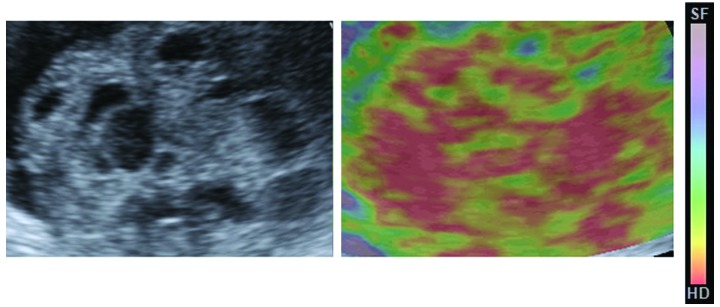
Conventional sonogram and elastogram from a pre-neoadjuvant chemotherapy patient with high-grade serous ovarian carcinoma, which appears predominantly yellow and green, and is clearly distinguishable. The case was scored as 2 on the four-point elasticity scale.
